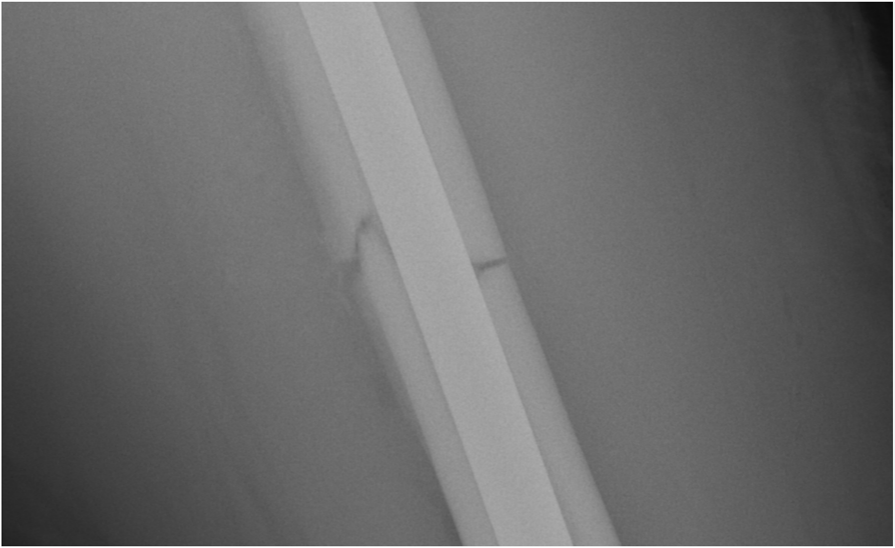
Radiographic example for CN difference < 1 mm
